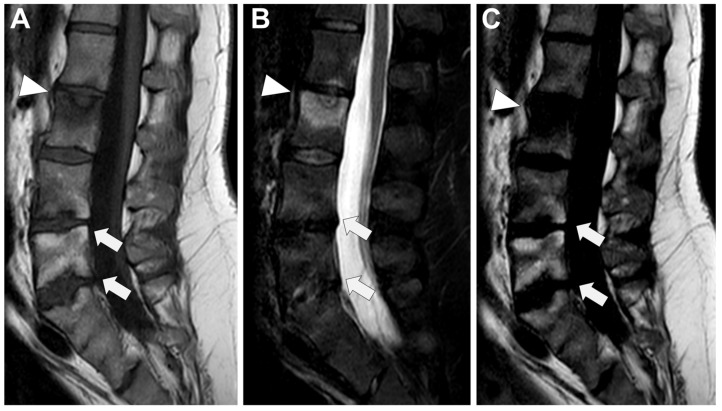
A 47-year-old male with a medical history of ankylosing spondylitis presented with the resurgence of upper lumbar pain with an inflammatory schedule. An MRI was performed, including (A) T1-weighted imaging (WI), (B) Dixon T2-WI with the Water Image (B), and the Fat image (C). This examination exhibits Andersson lesions of various ages. The white arrowhead shows the most recent lesions with a deep erosion in the middle of the upper L2 endplate with marked edema of the upper half of the L2 vertebral body. The white arrows show older lesions at the L4-L5 and L5-S1 levels with fatty replacement of the subchondral bone of the endplates.
